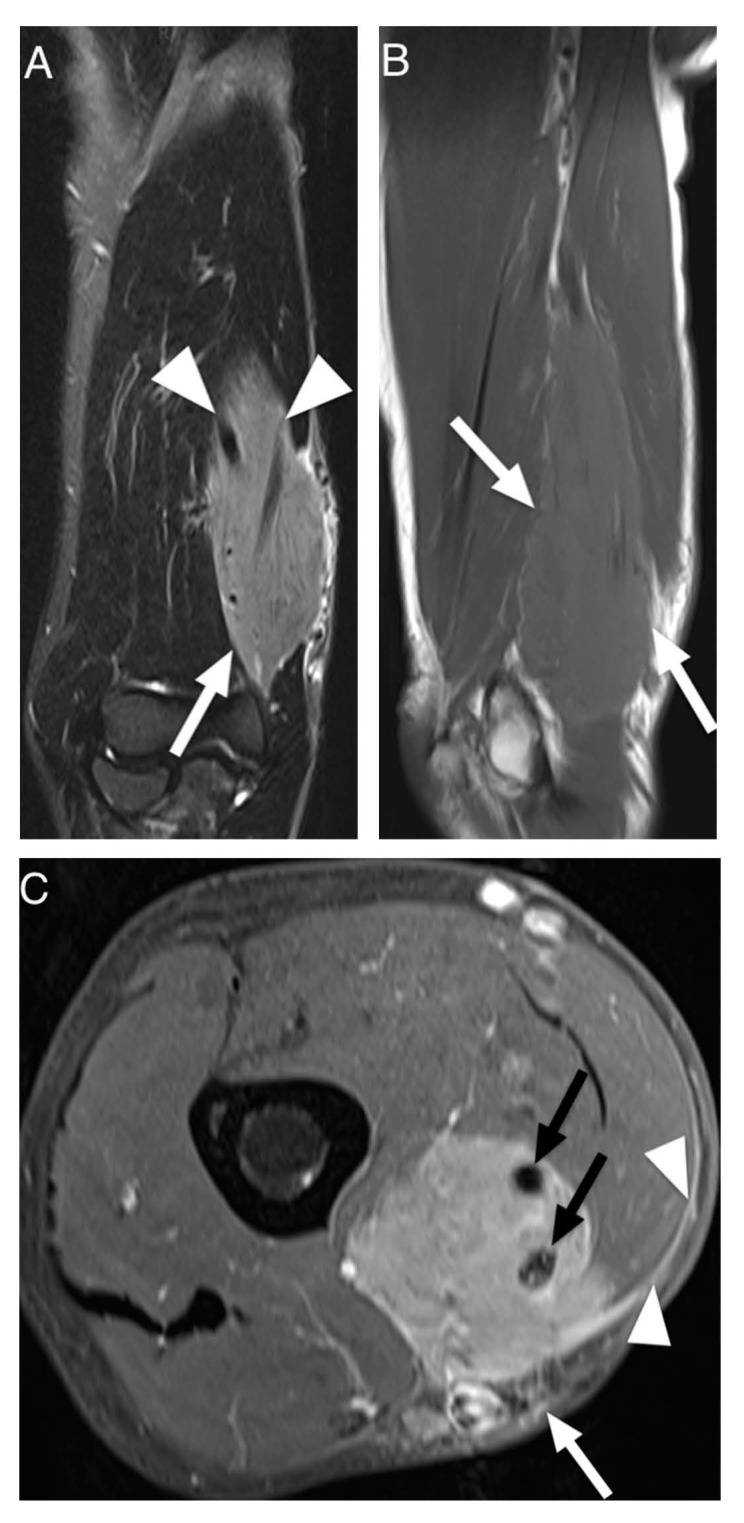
MRI of a 73-year-old man presenting with non-Hodgkin lymphoma manifestation in the flexor compartment of the upper arm. (A). Coronal T2-weighted TIRM image shows tumor of hyperintense signal intensity compared to muscle (arrow) and growth along the brachial neurovascular bundle (arrowheads). (B). Sagittal T1-weighted image shows long segmental involvement (arrows) and slightly hyperintense signal intensity of tumor in comparison to adjacent skeletal muscle. (C). Transversal contrast enhanced fat-saturated T1-weighted image shows homogenous contrast-enhancement of the lymphoma with encasement of the brachial neurovascular bundle (black arrows). Furthermore, subcutaneous stranding (white arrow) and contrast enhancement of the deep peripheral fascia (arrowheads) can be seen.
